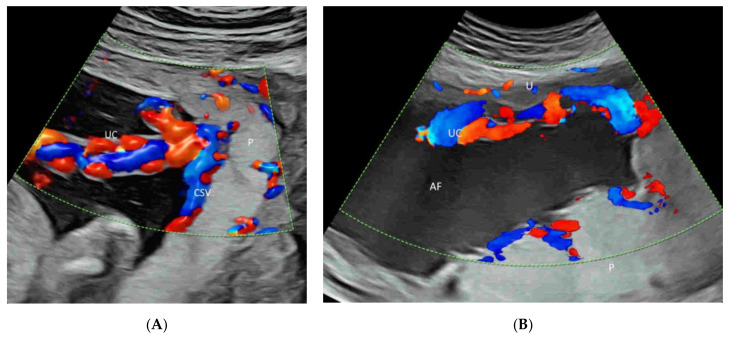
Color Doppler of the placenta showing a marginal (A) and velamentous (B) insertion of the umbilical cord (UC) to the placental (P). AF, amniotic fluid; CSV, chorionic surface vessels; U, uterus.
